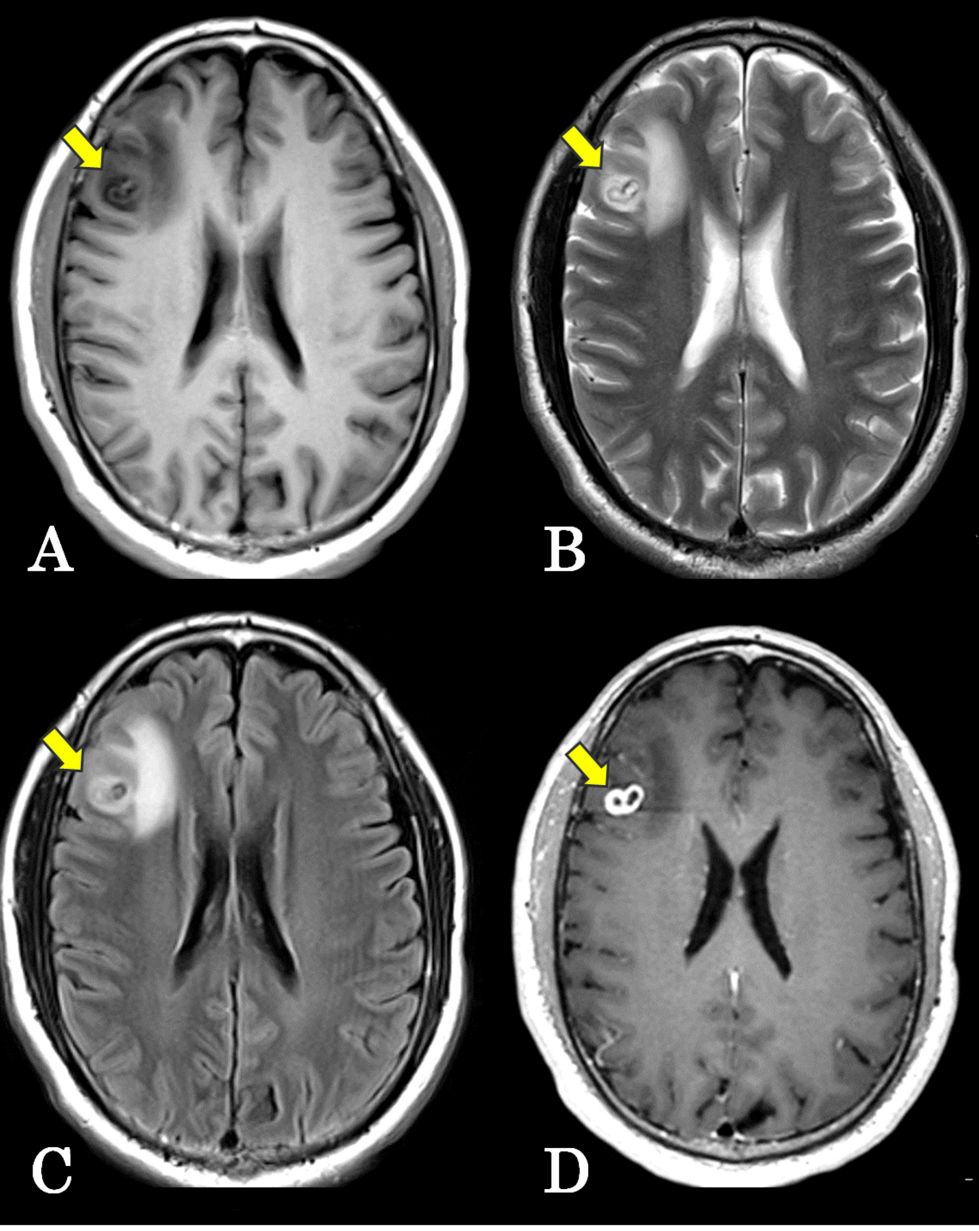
Magnetic resonance imaging (MRI) findings of the intracerebral lesion (A) T1-weighted MRI showing a hypointense lesion in the right frontal lobe. (B) T2-weighted MRI demonstrating marked perilesional edema. (C) Fluid-attenuated inversion recovery MRI showing prominent perilesional edema. (D) Gadolinium-enhanced T1-weighted MRI revealing a solitary cystic intracerebral lesion with ring-like enhancement. The arrows indicate the lesion.
